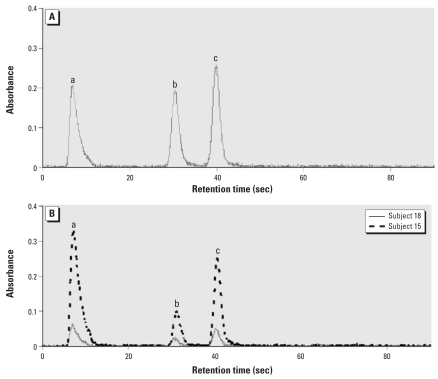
HG-CT-AAS analysis of As standards and As species in BECs showing separation and detection of arsine (a), methylarsine (b), and dimethylarsine (c) generated from a mixture of As standards, iAs^V^, MAs^V^, and DMAs^V^ (0.5 ng As each), spiked into a HepG2 cell lysate (*A*) and from BECs isolated from two subjects included in this study (*B*). The mixture of standards and BEC lysates were treated with cysteine to allow arsine generation from both trivalent and pentavalent As species.
